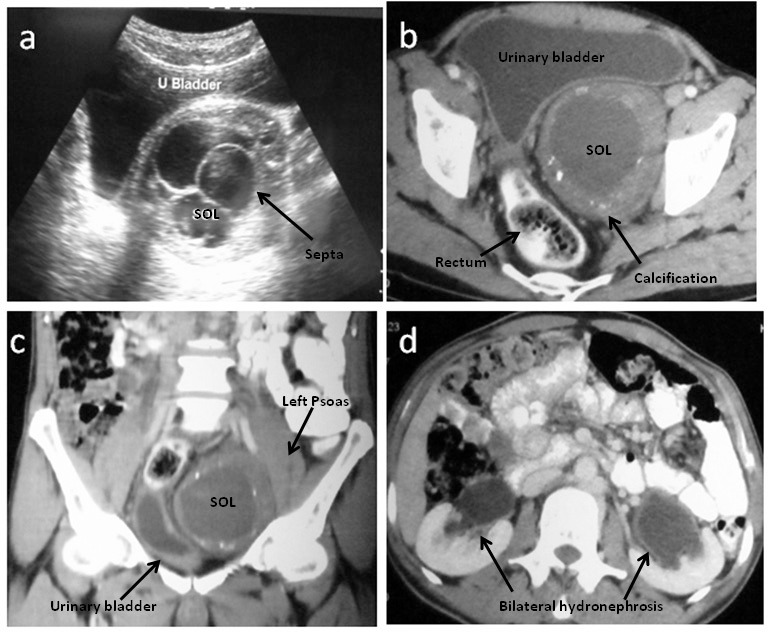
Cystic schwannoma imaging: Ultrasonography of the urinary bladder showed the cystic space occupying lesion (SOL) with multiple septations (a). Computed tomography of the abdomen showed a well defined cystic SOL (11cm × 8cm × 7cm) with multiple areas of focal calcification in the wall. The bladder and the rectum were pushed to the right (b). Coronal sections demonstrated the SOL to be intricately related to the left psoas muscle (c). There was bilateral hydroureteronephrosis (d).
